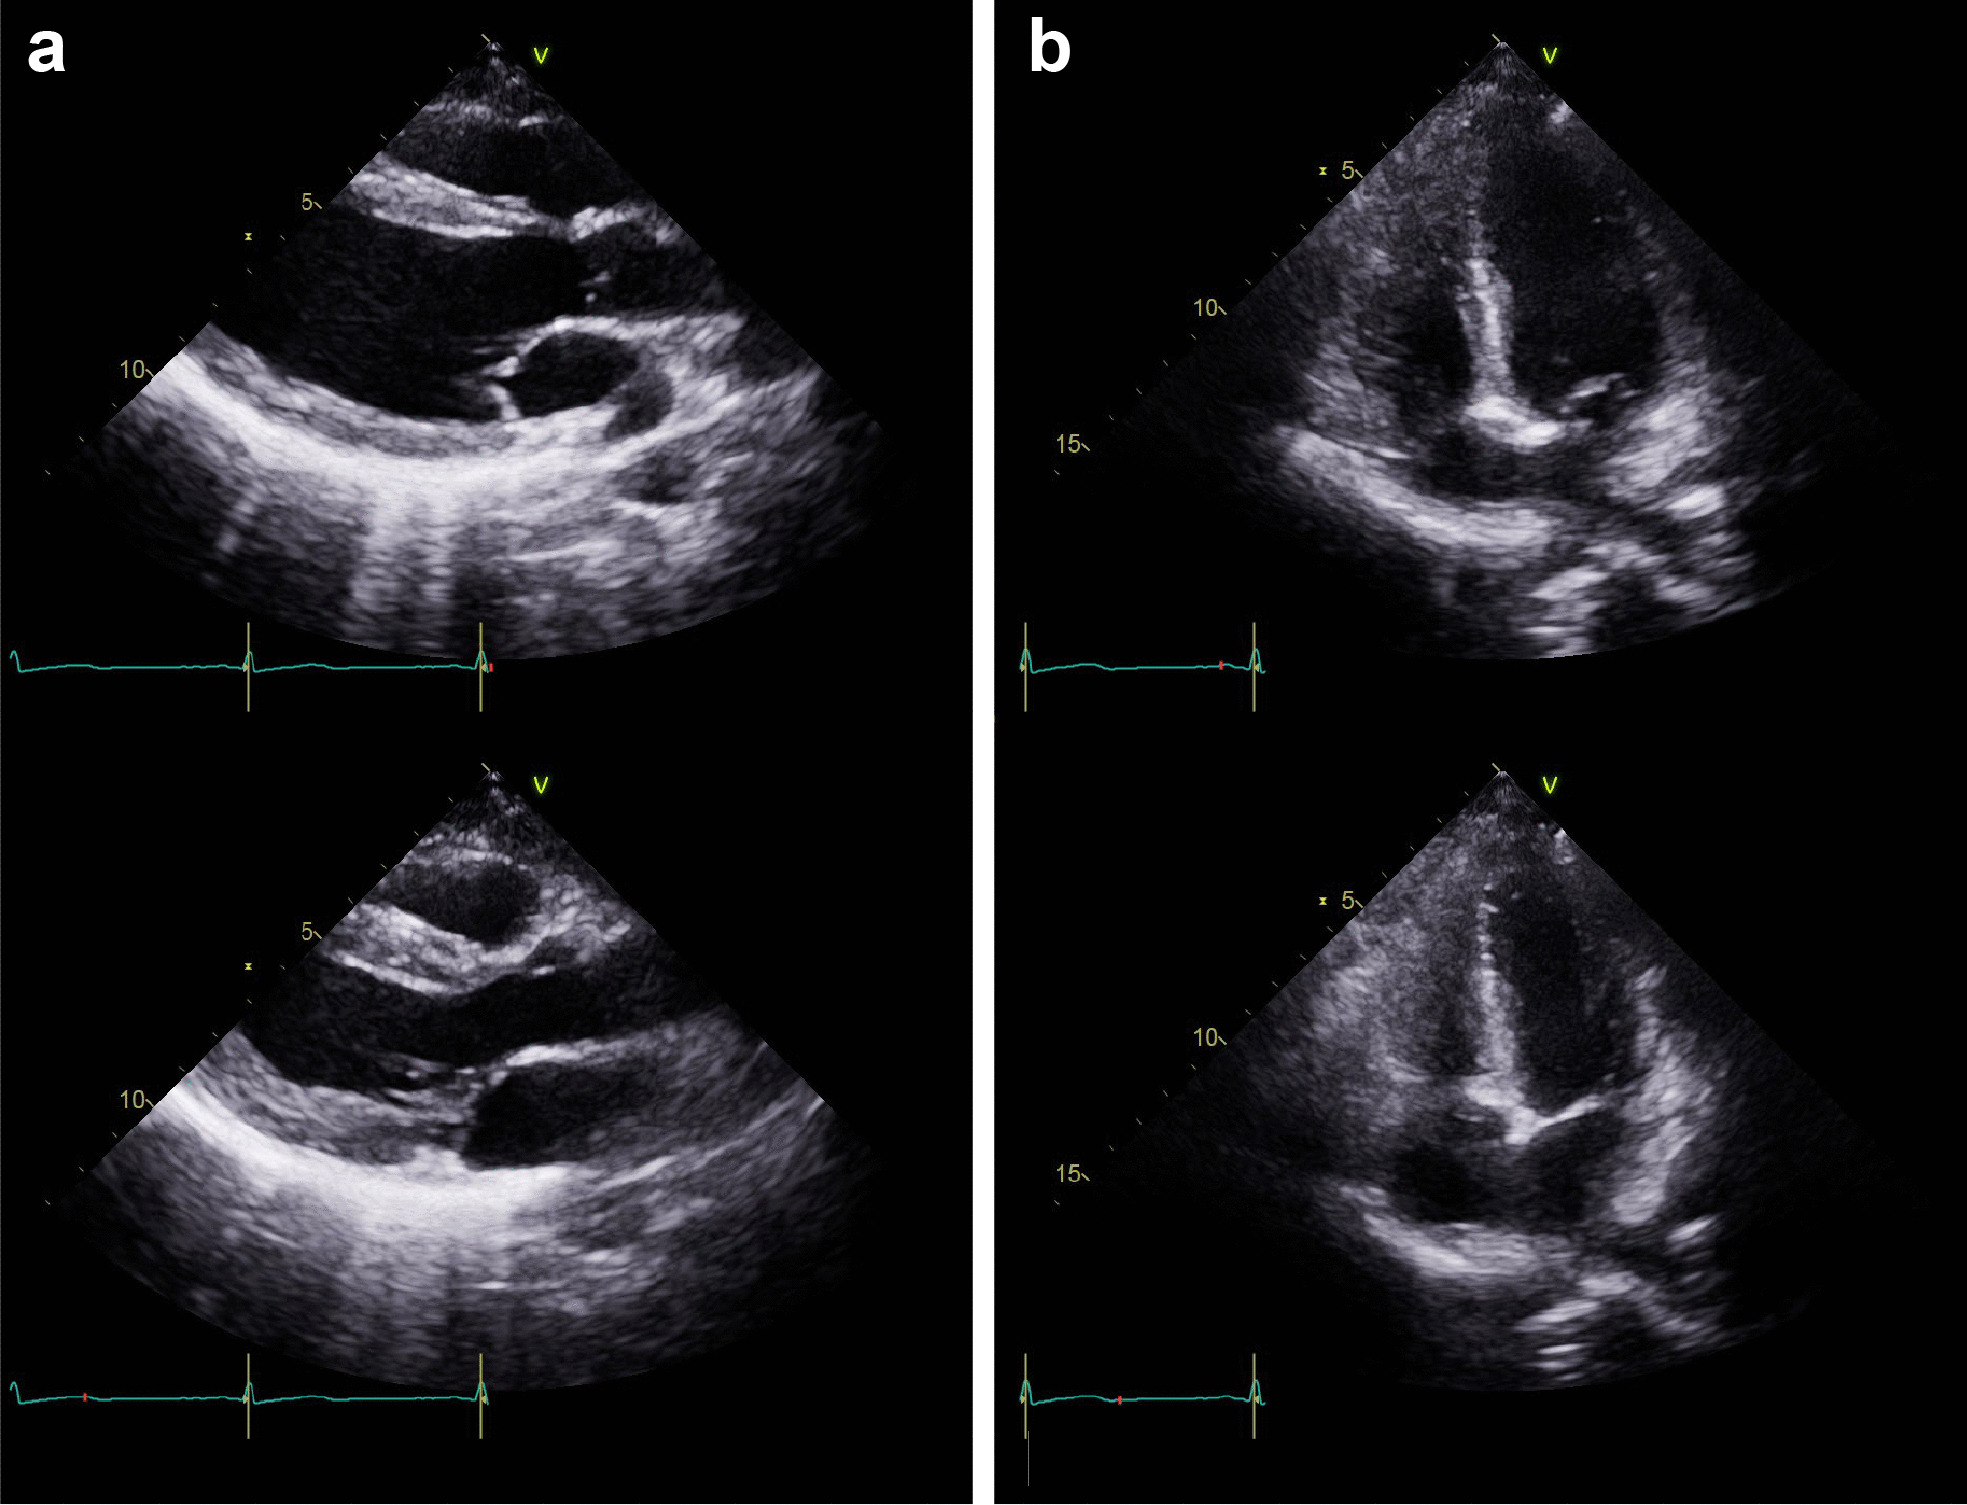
Follow up transthoracic echocardiogram at 5 days later of end-diastolic and end-systolic phase. Full recovery with normalized ejection fraction and no regional wall motion abnormality are observed. a Parasternal long axis view of end-diastolic (upper) and end-systolic phase (lower). b Apical four chamber view of end-diastolic (upper) and end-systolic phase (lower)
